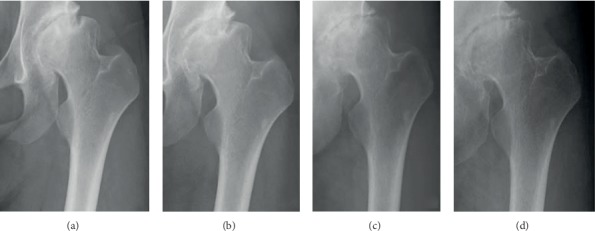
Plain radiograph for case 1, showing the hips at (a) first admission and at (b) 1 year, (c) 3 years, and (d) 4 years after the initiation of jiggling exercise.
